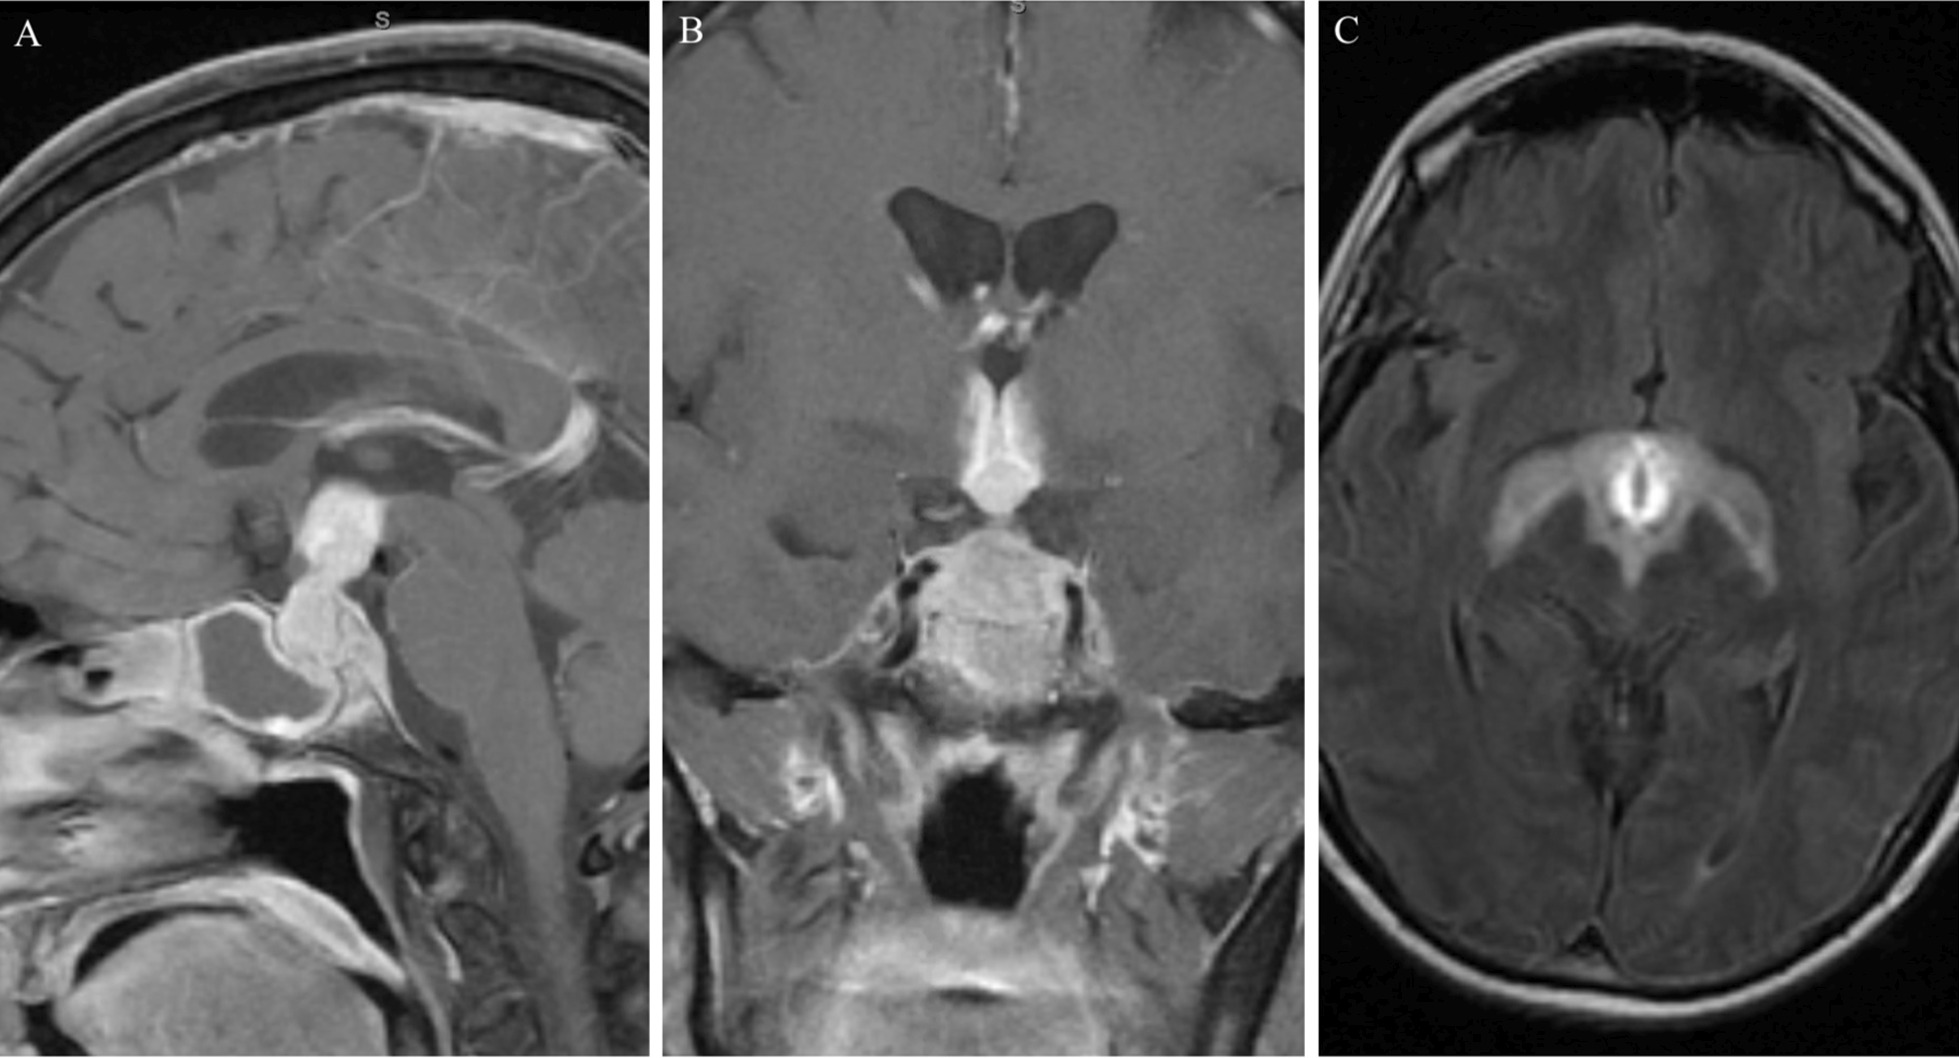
MRI brain with and without gadolinium 1 month after initial presentation. Sagittal T1 post-gadolinium (A). Coronal T1 post-gadolinium (B). Axial T2 FLAIR C
